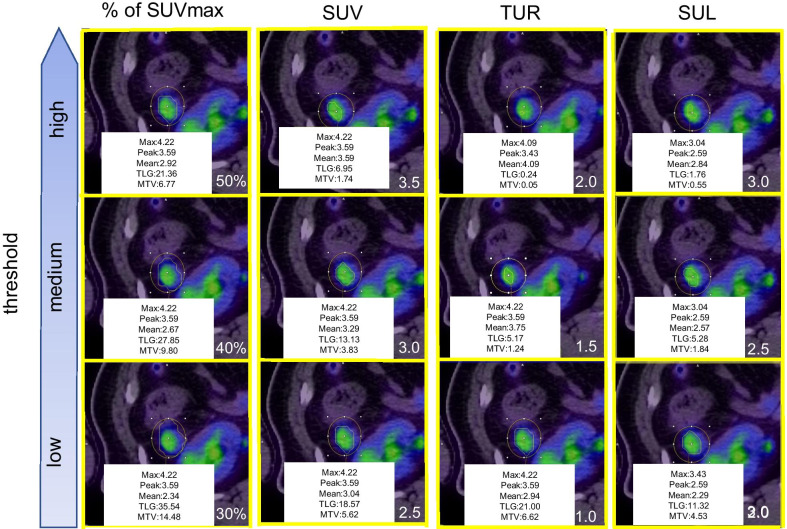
Thresholds in case of a lower SUVmax tumor. SUVmax: maximum standardized uptake value, SUV peak: peak standardized uptake value, SUV mean: mean standardized uptake value, MTV: metabolic tumor volume, TLG: total lesion glycolysis, TUR: tumor-to-liver uptake ratio, SUL: SUV normalized to lean body mass; VOI: volume of interest. VOIs for TUR2 were smaller than in other thresholds. VOIs in % of SUVmax were larger than in other thresholds. For each threshold, the lower the value, the larger the VOI
